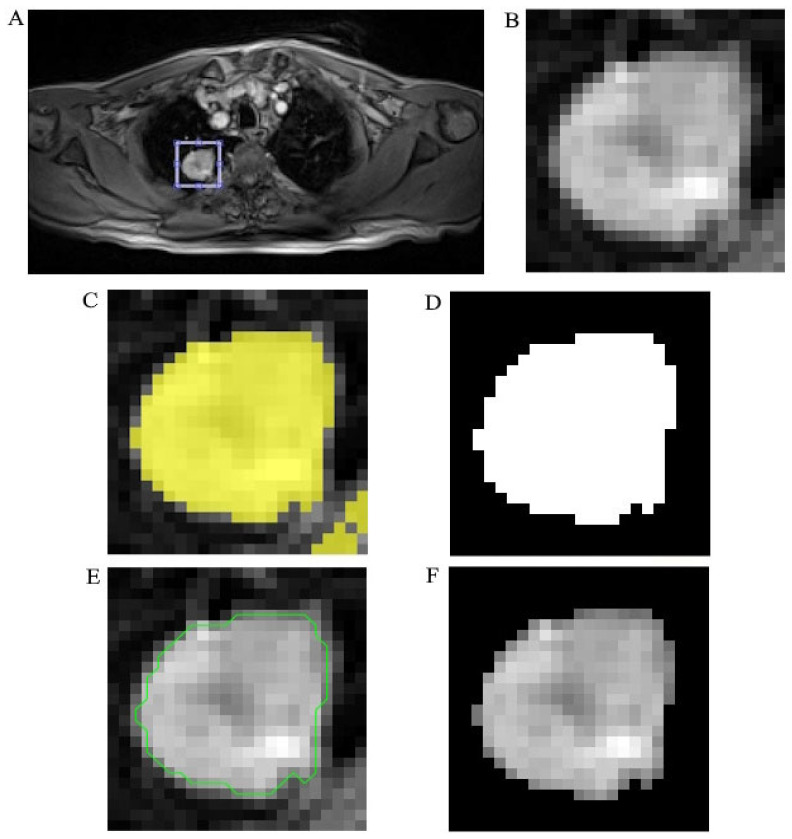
The segmentation process of MR image. (A) Non-small cell lung cancer (NSCLC) localization on input MR image. (B) Delineation of the region of interest (ROI). (C) Thresholding and binarization. (D) Resulting binary masks. (E) Contour enhancing (green line). (F) Final segmented ROI exporting.
